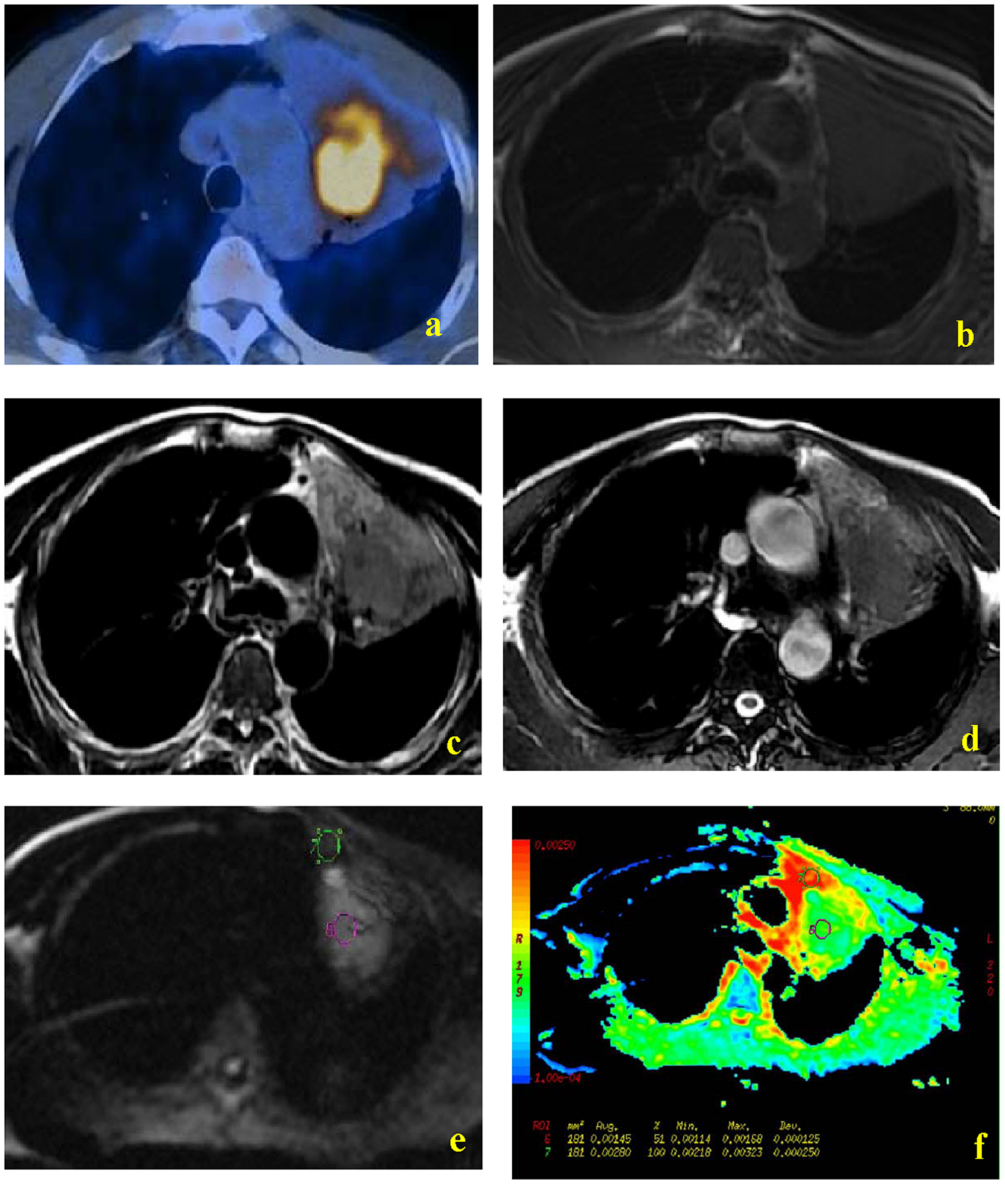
MR and PET/CT images of a 39-year-old woman with lung adenocarcinoma. PET-CT indicated greater uptake of FDG by tumor than atelectasis (a). T1W MR imaging showed a soft-tissue shadow in the left upper lobe, but similar signal intensities of the central lung carcinoma and the distal atelectasis were noted (b). FRFSE T2W images and FIESTA images indicated hypointensity of the tumor mass relative to the atelectasis (c and d, respectively). DW images obtained with a diffusion gradient of 500 s/mm^2^ allowed easy differentiation of tumor and atelectasis (e and f).
